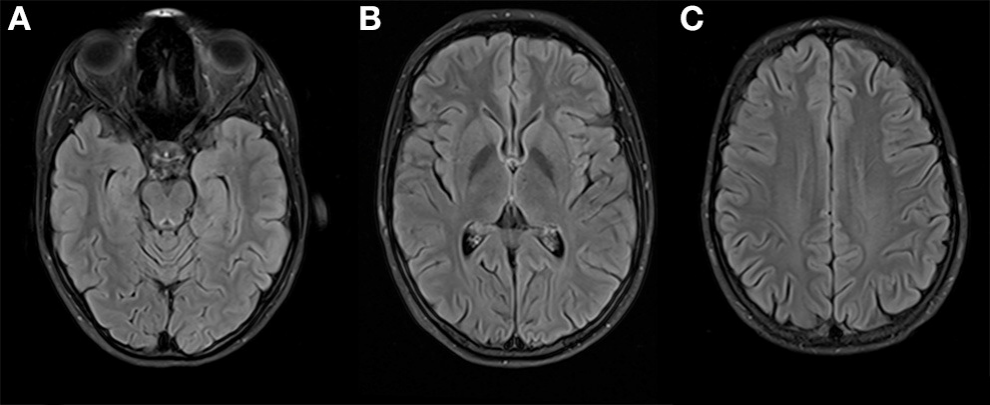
Brain imaging at 3 Tesla MRI (T2 FLAIR weighted) on three different levels (A–C) shows no suspicious signal alterations, especially on both mesial temporal lobes (A) and insula (B).
